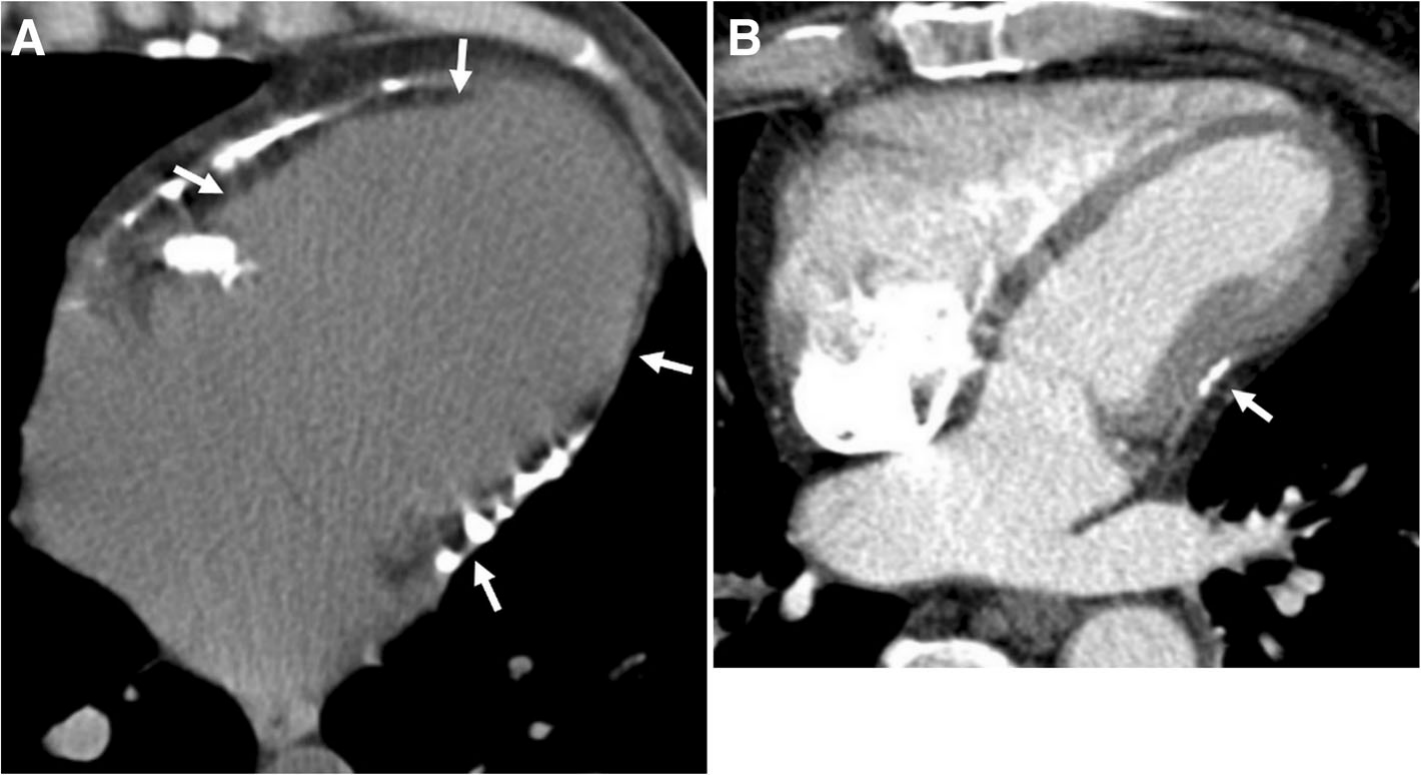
A 35-year-old man underwent to CT scan due to decreased cardiac output. a Axial CT image reveals entrapped heart appearance (arrows) with diffuse pericardial calcification which is compatible with constrictive pericarditis. b Axial contrast-enhanced CT image following surgery demonstrates diminished compressive effect to the right side of the heart; however, residual compressive effect to the left ventricle (arrow) was still evident despite surgery
